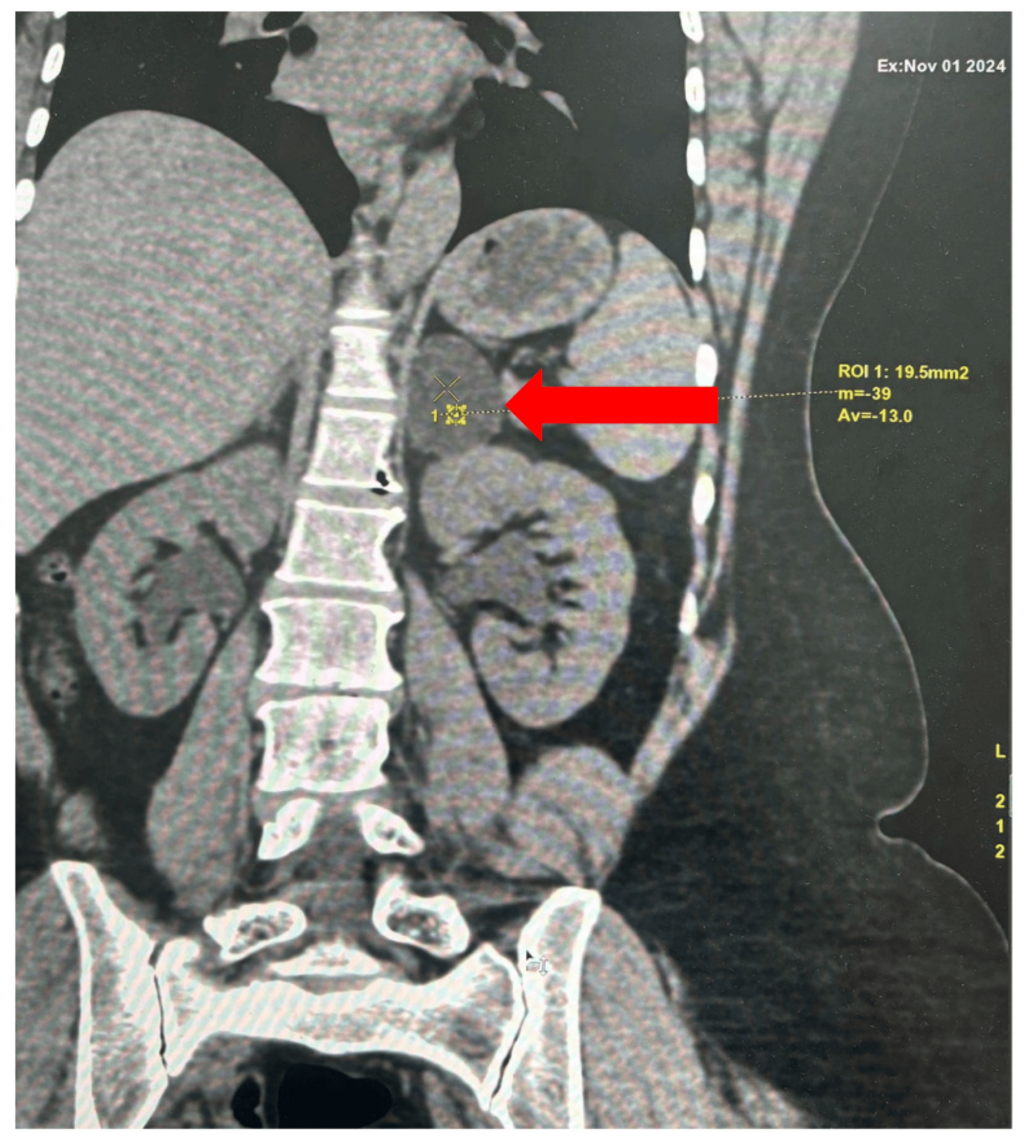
Adrenal computed tomography showing left adrenal mass of unenhanced attenuation of -13 HU (red arrow)
